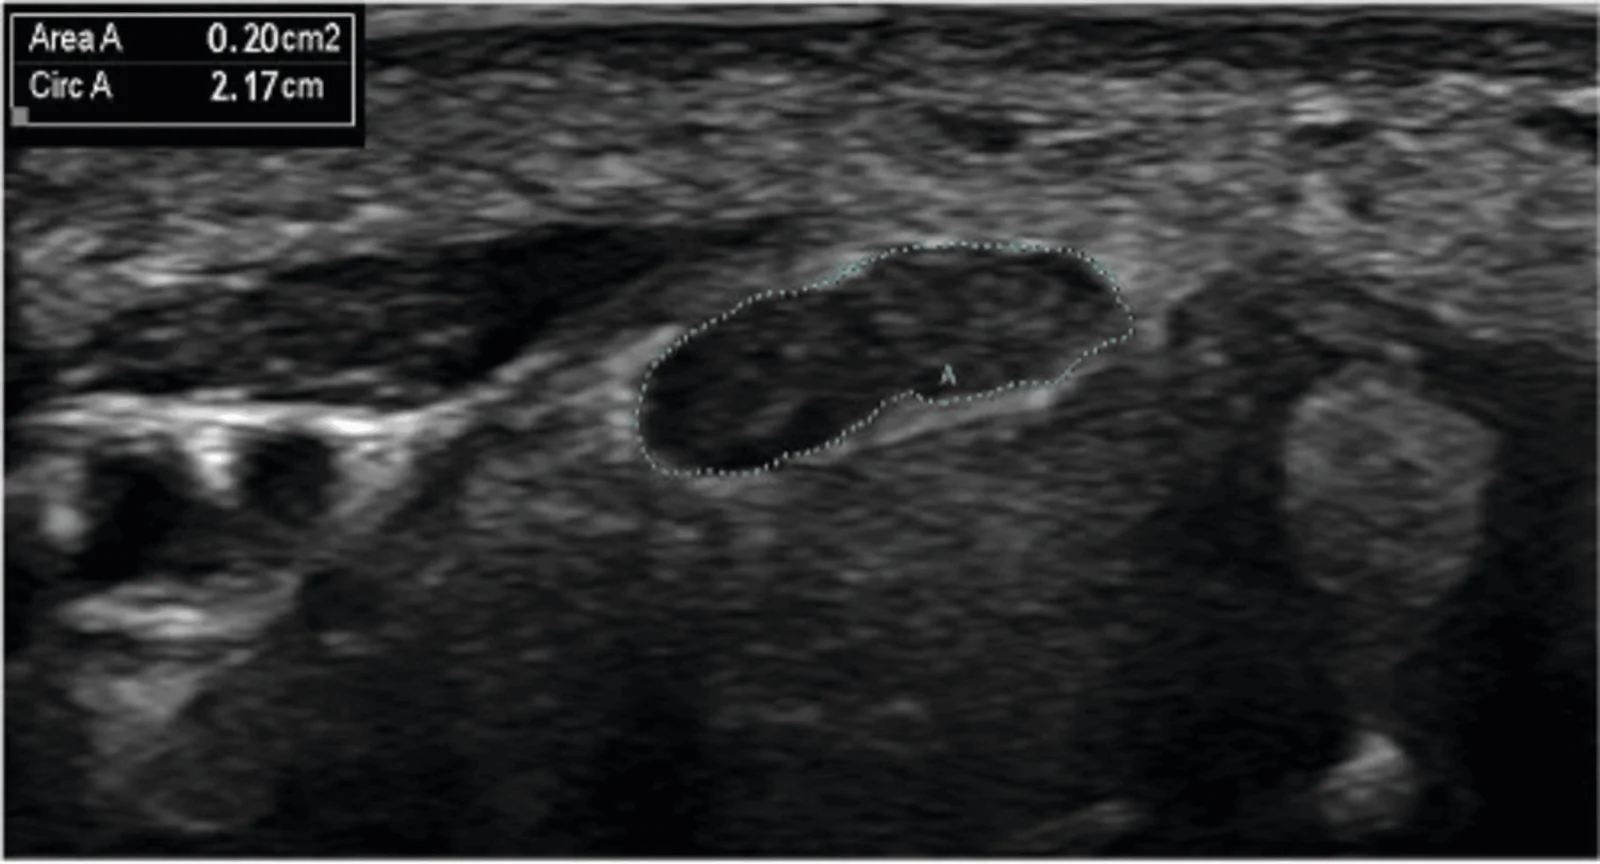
Ultrasonographic measurement of the median nerve cross-sectional area (CSA) at the carpal tunnel inlet. The dotted outline represents manual tracing of the median nerve for CSA measurement, an established parameter for evaluating carpal tunnel syndrome.
